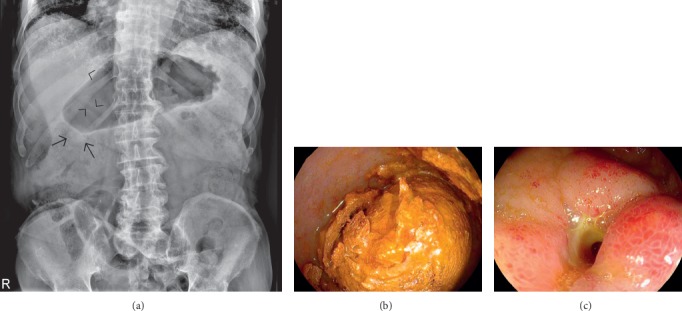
Supine abdominal radiograph (a) in a case of Bouveret's syndrome demonstrates pneumobilia (arrowheads) and a dilated stomach. An ectopic slightly radiopaque stone in the right upper abdominal quadrant was originally missed (arrows). Esophagogastroduodenoscopy in the same patient reveals an impacted large gallstone in the duodenal bulb (b). The fistulous stoma is well visualized in (c).
